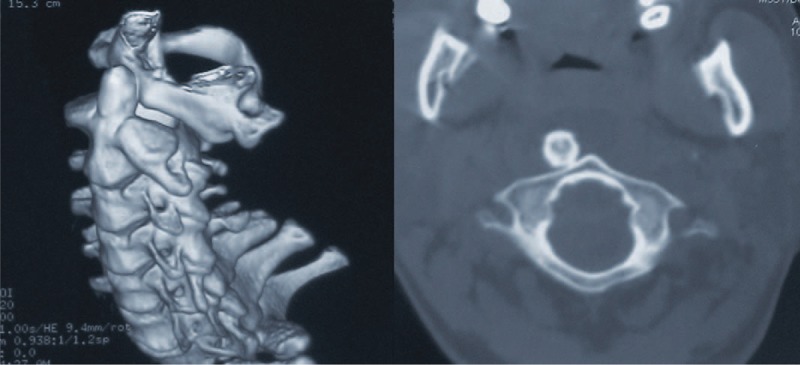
Axial CT scans and 3D reconstructions revealed intact odontoid process lied ventral to the anterior arch of atlas. 3D = three-dimensional, CT = computed tomography.
